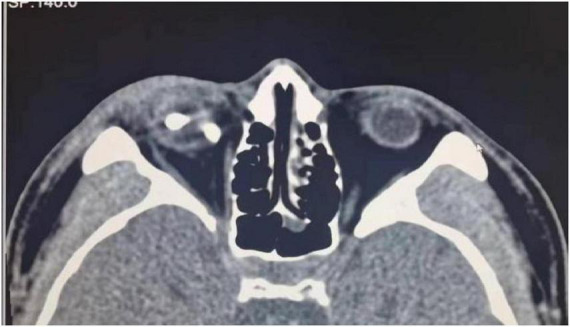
Thirteen days post-surgery, a follow-up computed tomography (CT) scan revealed that some intraocular and intraorbital foreign bodies remained.
